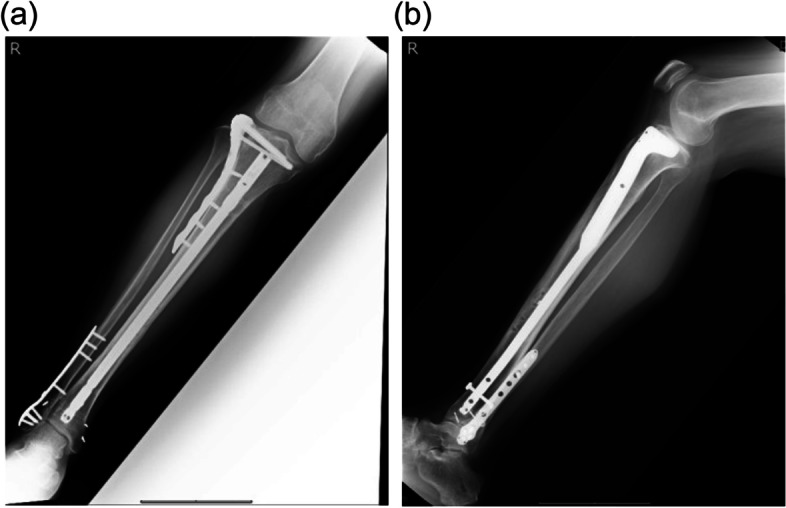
a Frontal and b lateral X-rays of the right tibia after bone fixations
